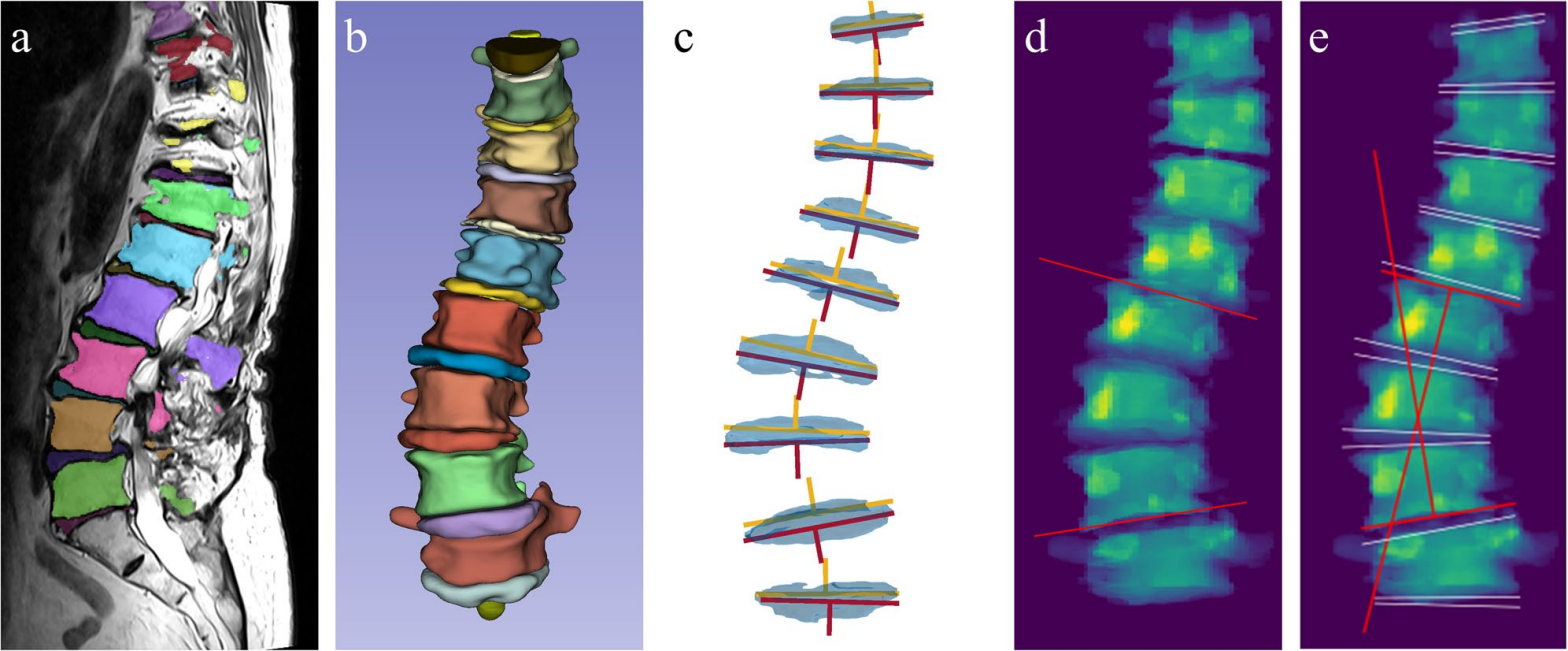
Visualization of the different steps of the Cobb angle algorithm. a Automatically generated segmentation visualized on the sagittal MR image of the lumbar spine. b Same segmentation visualized in 3D in the coronal plane. c Coronal view of all intervertebral discs with the upper (yellow) and lower (red) planes shown as a 2D line and the normal vector. d Example of a manual measurement on the 2D coronal projection image. e Visualization of the automatic measurement on the 2D coronal projection image
